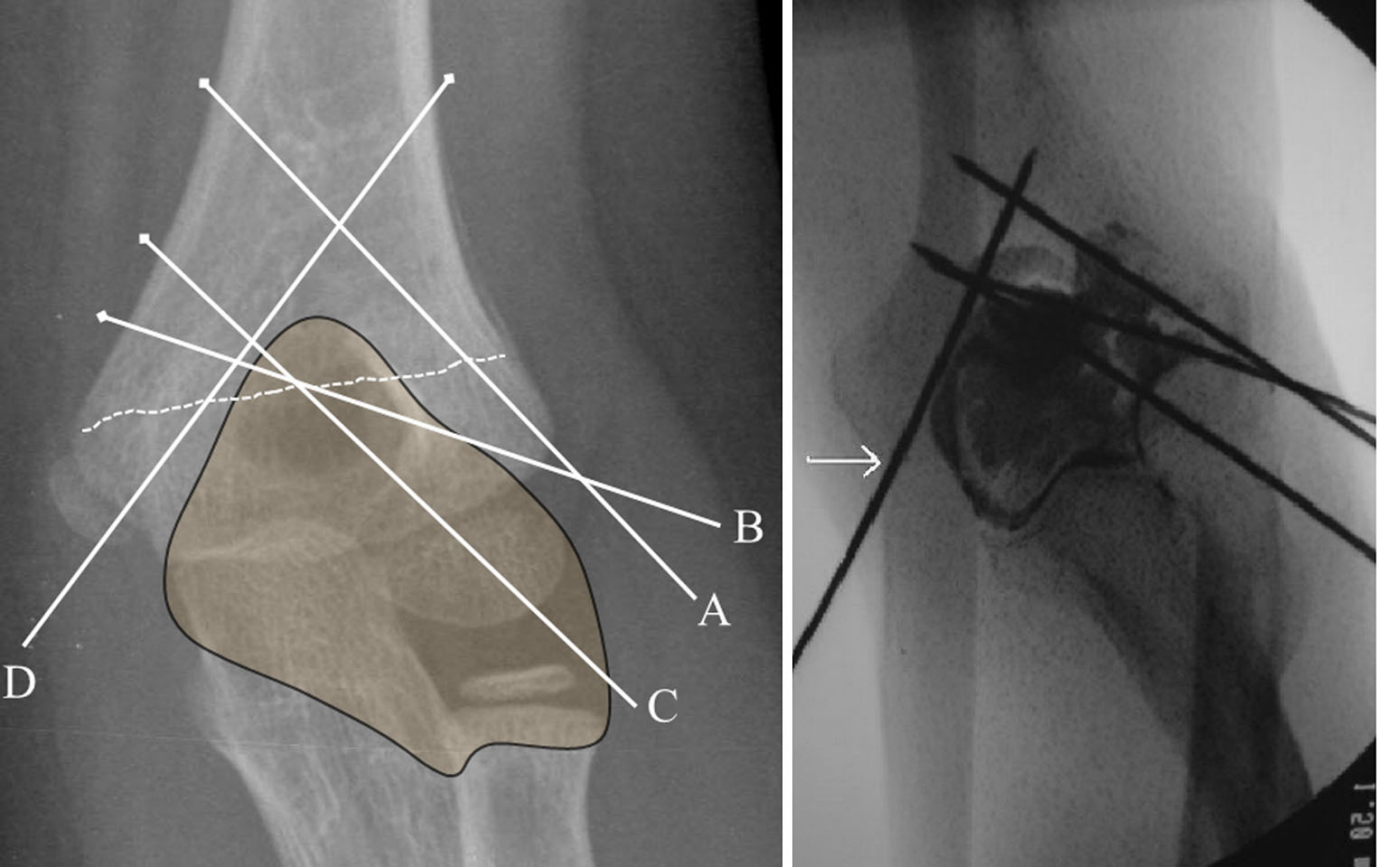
Anteroposterior radiograph demonstrating the relationship of the pins to the capsule. The arthrogram shows that the medial pin (*white arrow*) is extracapsular. Laterally,* pins A * and* B* appear to be extracapsular, and* pin C* appears to be intracapsular
